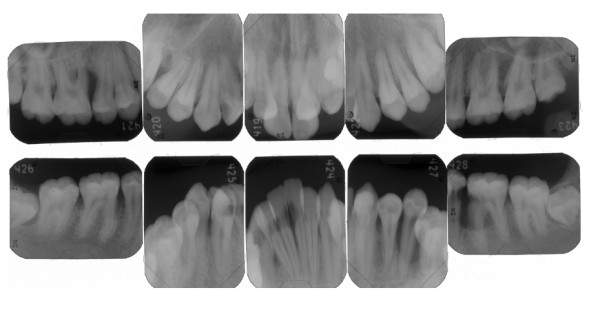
Baseline full-mouth periapical radiograph.
